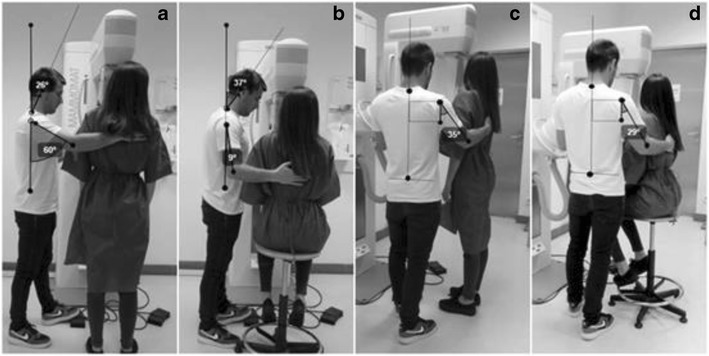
CC breast positioning: a and c radiographer postures without intervention; b and d alternative postures when the radiographer and patient have identical statures
